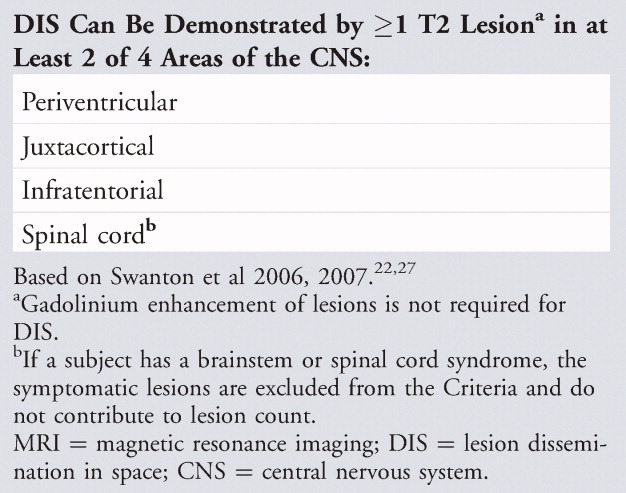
#### Magnetic Resonance Imaging Criteria for DIT
